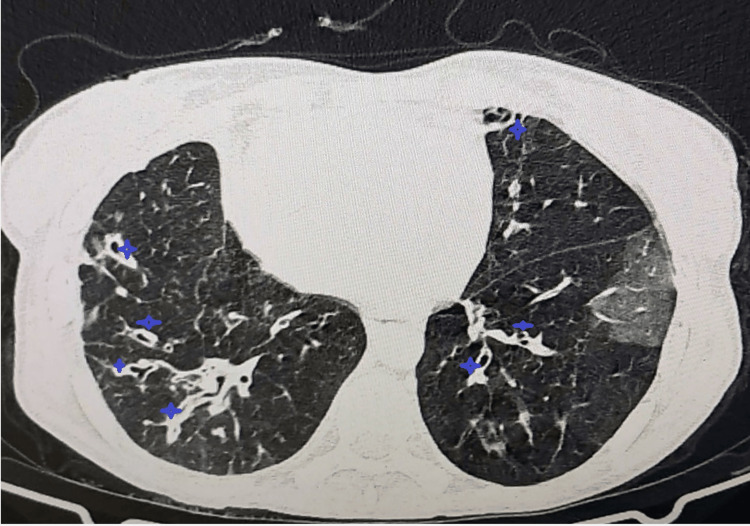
Case 4: Chest CT scan, axial section in parenchymal window, showing extensive pneumonic consolidations in a patient with common variable immunodeficiency, associated with bilateral bronchiectasis.
